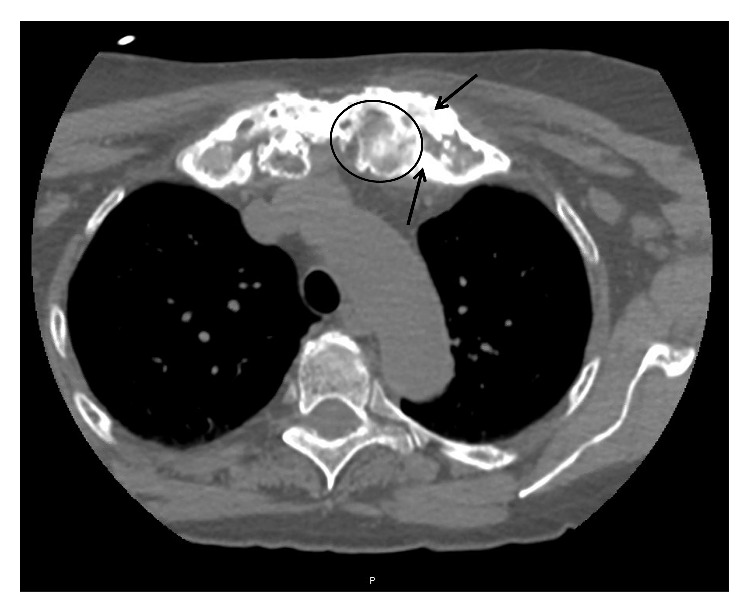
CT biopsy imaging axial body view with a slice thickness of 2.00 demonstrating lytic (example inside circle) and sclerotic lesions (examples of thickened bone pointed to with arrows) of the left medial clavicle and left first rib.
